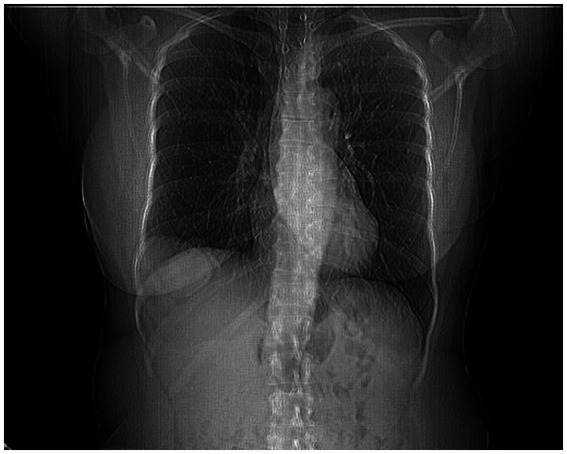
Coronal CT image showing right diaphragmatic hernia with herniation of the liver into the thoracic cavity.
